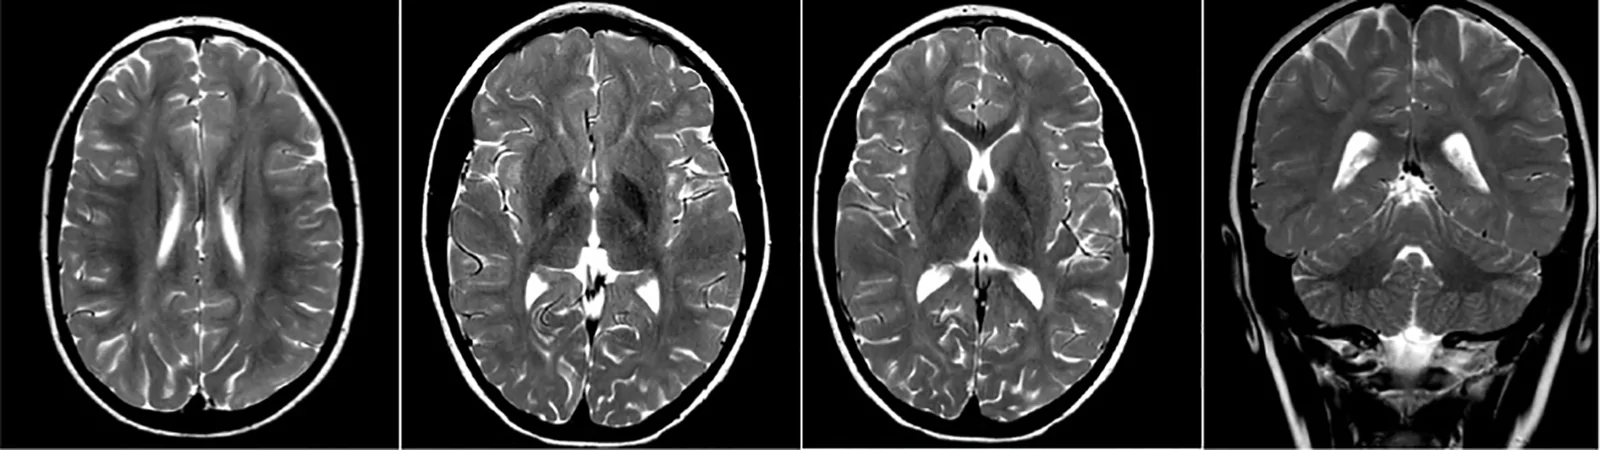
Brain magnetic resonance imaging findings. T2-weighted images showing diffuse white-matter hyperintensity involving the internal capsule and bilateral corona radiata, consistent with hypomyelinating leukodystrophy.
